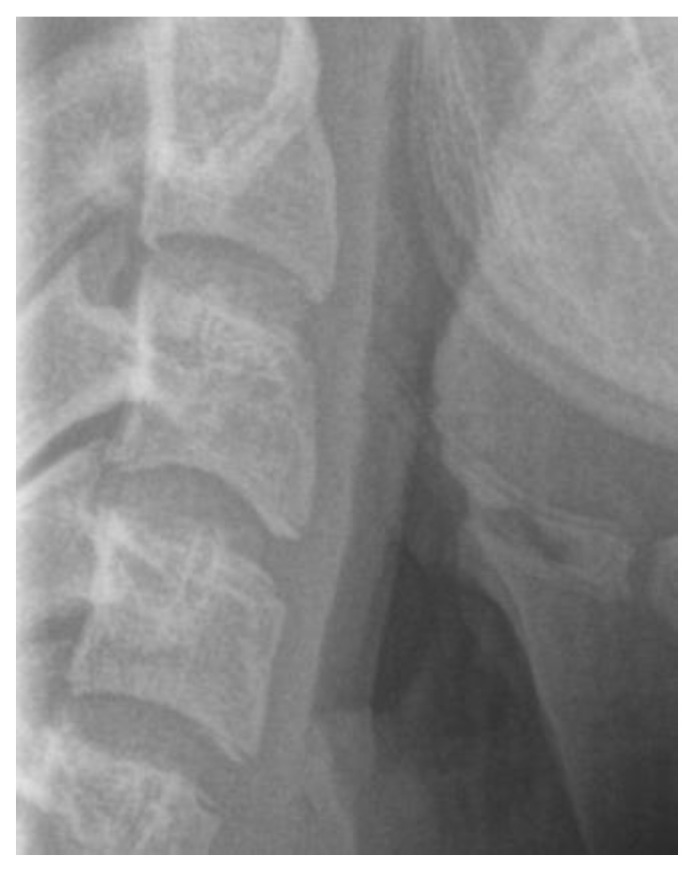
Cervical vertebrae radiograph—square shape.
